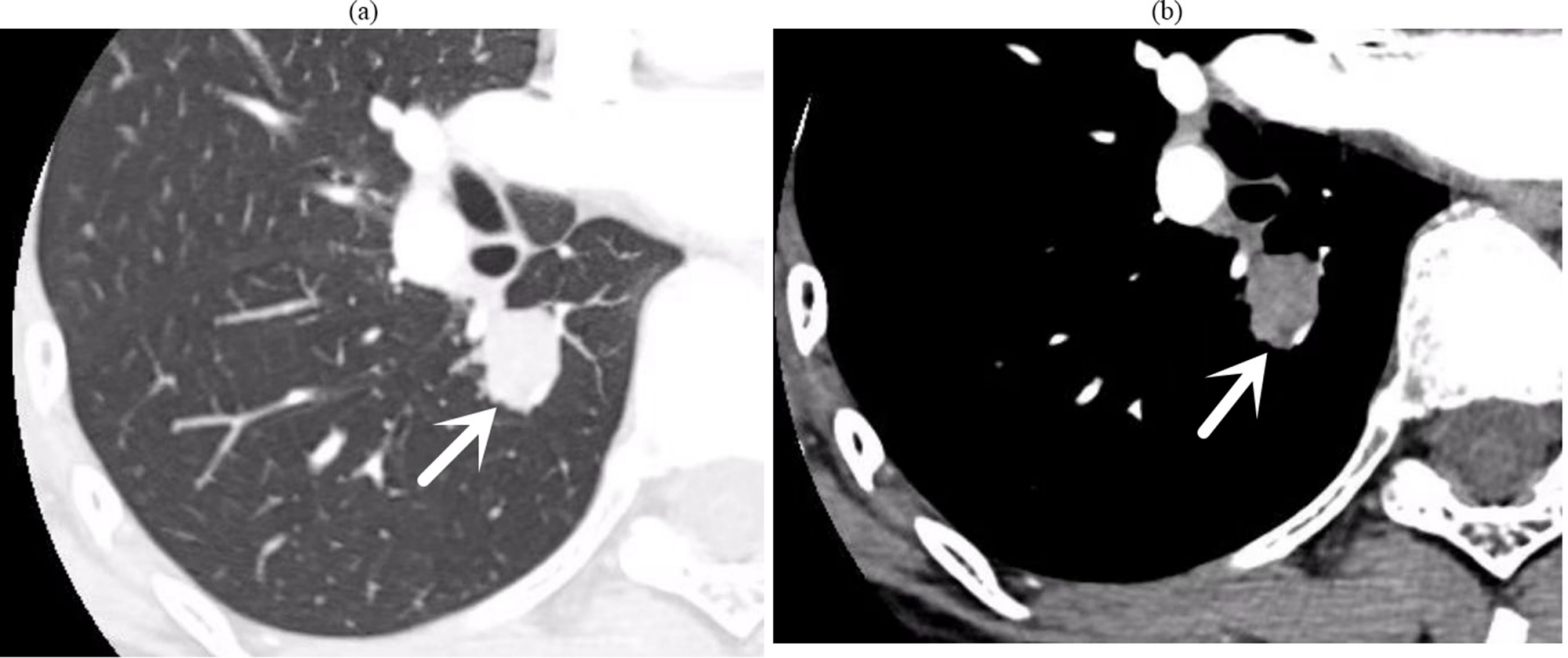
(a) (Lung window), (b) (mediastinal window) chest target-enhanced CT: small nodules in the dorsal segment of the right lower lobe, with punctate calcifications visible within it, surrounded by multiple punctate and nodular shadows; nodular growth was seen in the dorsal segment of the bronchus.
